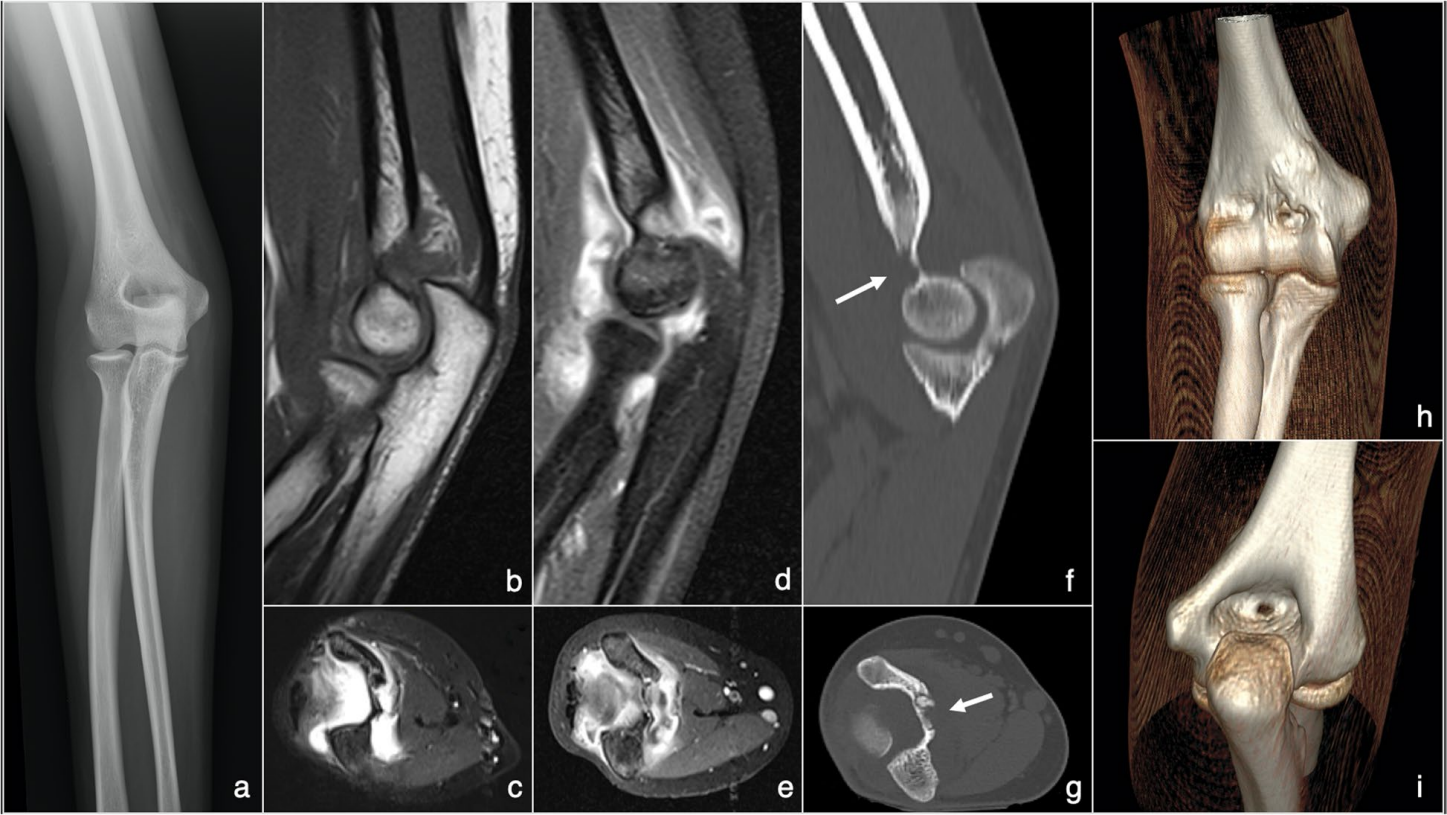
Various radiographic modalities performed on a patient with elbow osteoid osteoma presented in this study: a plain radiography anteroposterior projection image of the right elbow affected by osteoid osteoma; b sagittal MRI projection image of the right elbow showing mild signs of elbow oedema; c axial MRI projection image of the right elbow showing signs of elbow oedema; d sagittal magnetic resonance arthrography projection image of the right elbow showing signs of elbow oedema; e axial magnetic resonance arthrography projection image of the right elbow showing signs of elbow edema; f sagittal CT projection image of the right elbow with an arrow pointing to the osteoid osteoma site in between coronoid and olecranon fossa; g axial CT projection image of the right elbow with an arrow pointing to the osteoid osteoma site in between coronoid and olecranon fossa; h 3D reconstruction CT image of the right elbow demonstrating osteoid osteoma site from the anterior view; i 3D reconstruction CT image of the right elbow showing osteoid osteoma site from the posterior view
